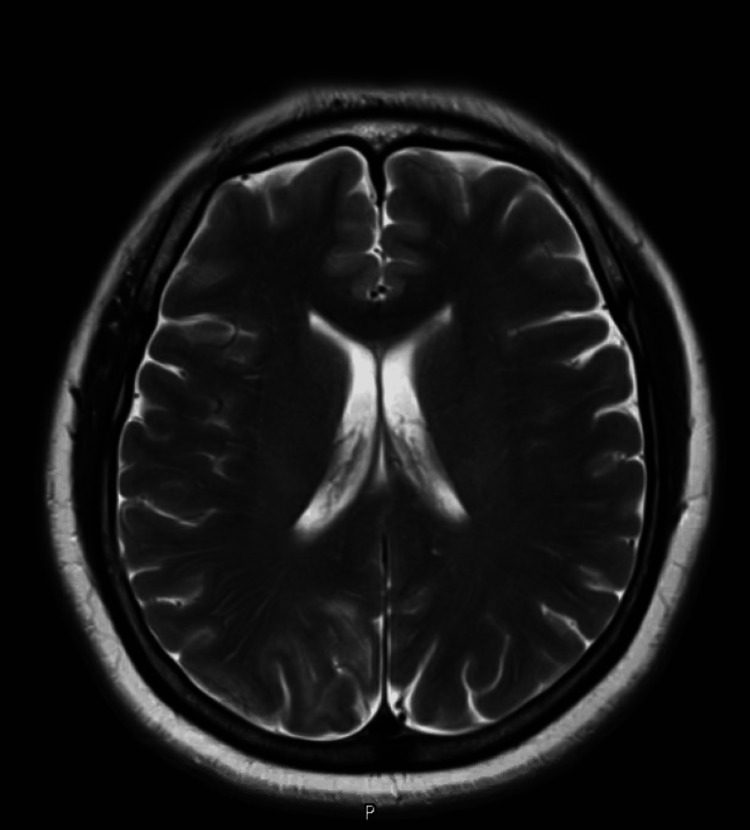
T2-weighted contrast-enhanced brain MRI showing significant regression of the previously noted right occipitoparietal lesion.
